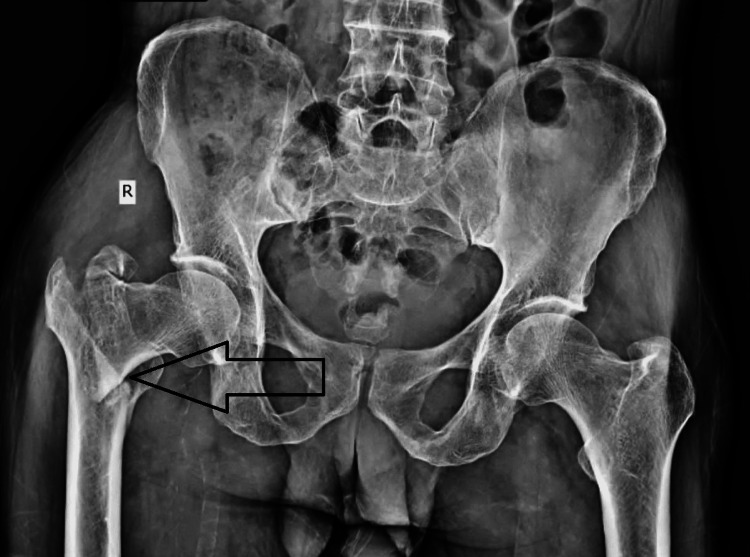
Pre-operative radiograph of the pelvis and both hips showed an intertrochanteric fracture of the right femur.
